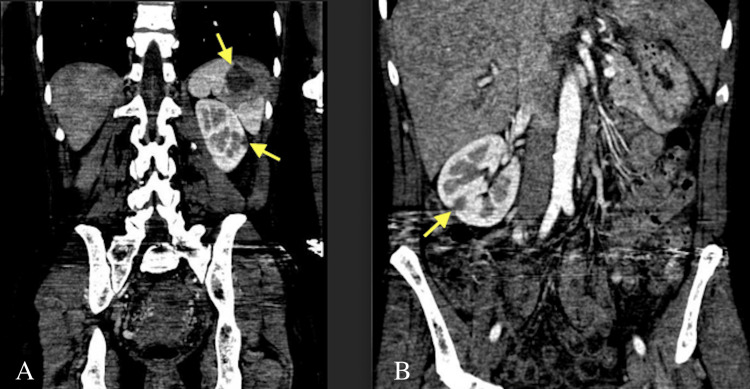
CT abdomen and pelvis coronal view performed on admission (A) Top arrow indicating hypodense splenic infarct, bottom arrow indicating hypodense left renal infarct. (B) Arrow indicating hypodense right renal infarct.
